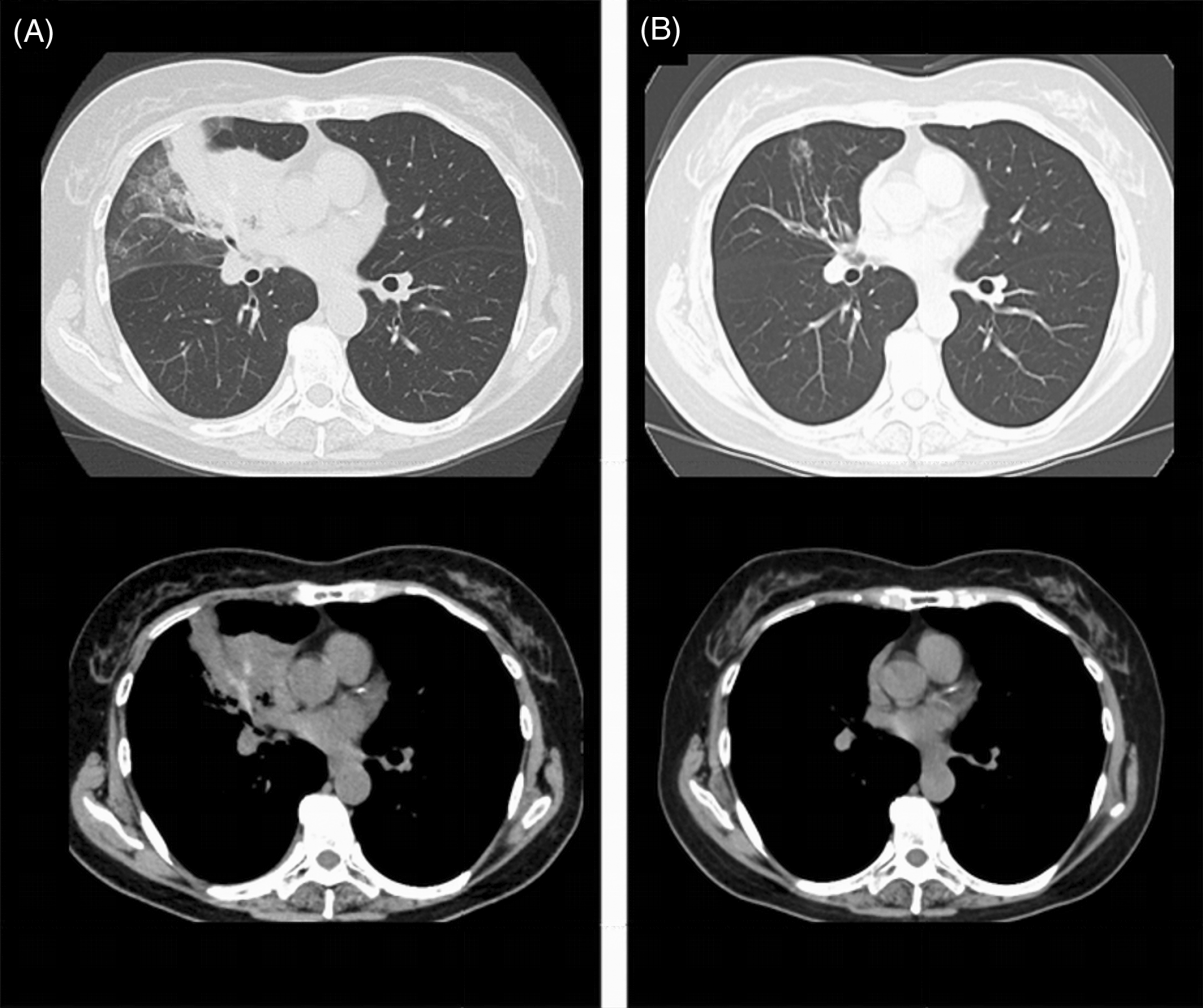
(A) Chest computed tomography showed infiltrative shadow in the right middle lobe and high‐attenuation mucus. (B) At 55 days of treatment, the infiltration shadow and mucous plug in the right middle lobe had resolved.
